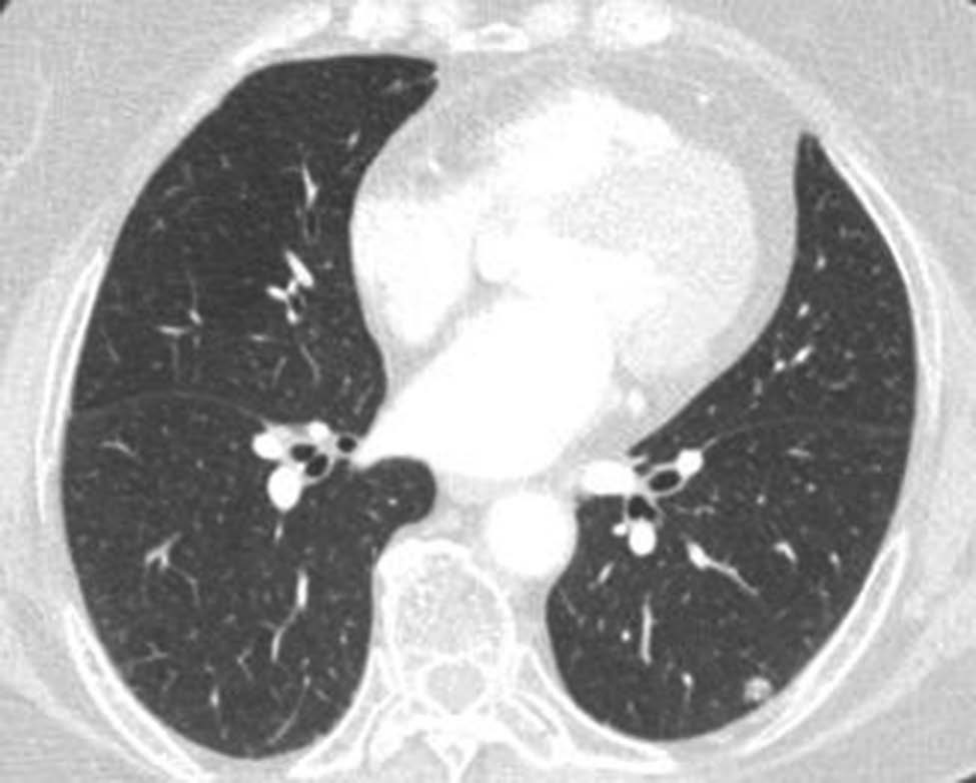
Female, aged 75 years old. Chest CT showed: mixed ground glass nodules in the outer basement segment of the lower lobe of the left lung, the maximum section diameter was about 7 mm * 7 mm, circular shape, clear boundary and marginal segmentation.
